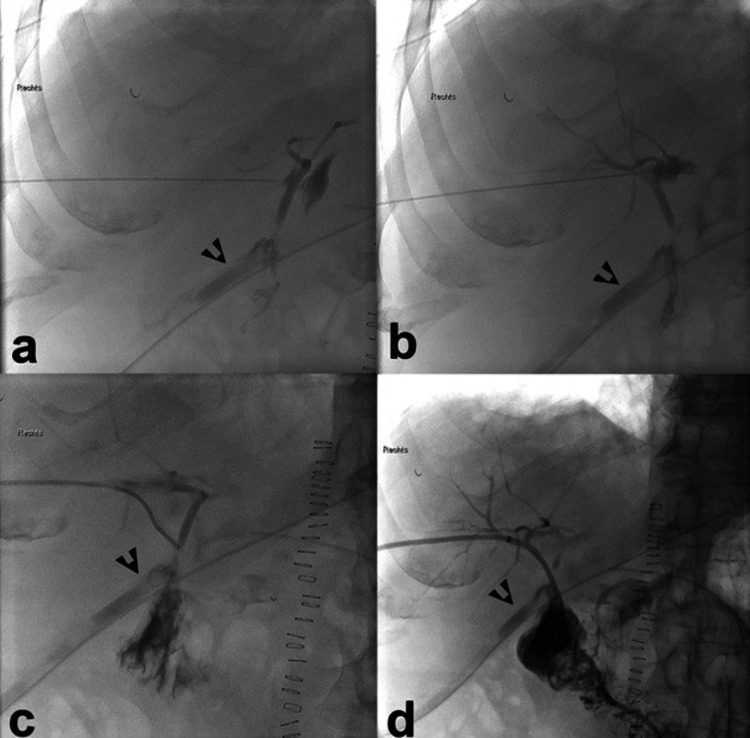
Percutaneous transhepatic biliary drainage (PTBD) placement following clinical suspicion of biliodigestive anastomotic insufficiency (bile output >300 ml/day via surgical drainage). a–b: After sonographically and fluoroscopically guided puncture of the right biliary system using a Neff set (Cook Medical, Bloomington, IN, USA), contrast injection reveals a clear leak from the common bile duct (CBD) with immediate extravasation into the surgical drainage (black arrowhead). c–d: Successful probing of the biliary tree using a 4F Berenstein catheter (Cordis, Miami Lakes, FL, USA) and a 0.035″ hydrophilic guidewire (Terumo, Tokyo, Japan), followed by insertion of an 8F pigtail-configured drainage catheter (Cook) over a stiff 0.035″ wire (Amplatzer Super Stiff, Boston Scientific, Marlborough, MA, USA). Final placement is confirmed, and the drain is secured subcutaneously.
